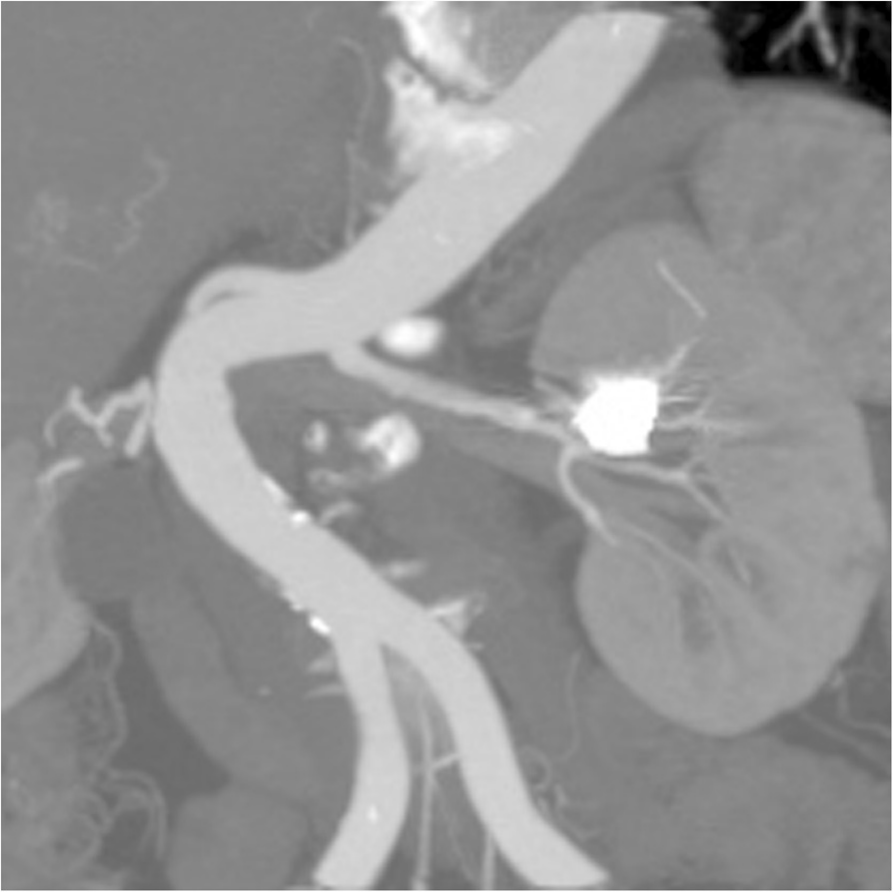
Abdominal contrast-enhanced computed tomography 1 month after the procedure. Oblique coronal multiplanar reconstruction clearly shows the aneurysm exclusion from complete coil filling and normal parenchymal contrast enhancement without evidence of segmental ischemic damage.
